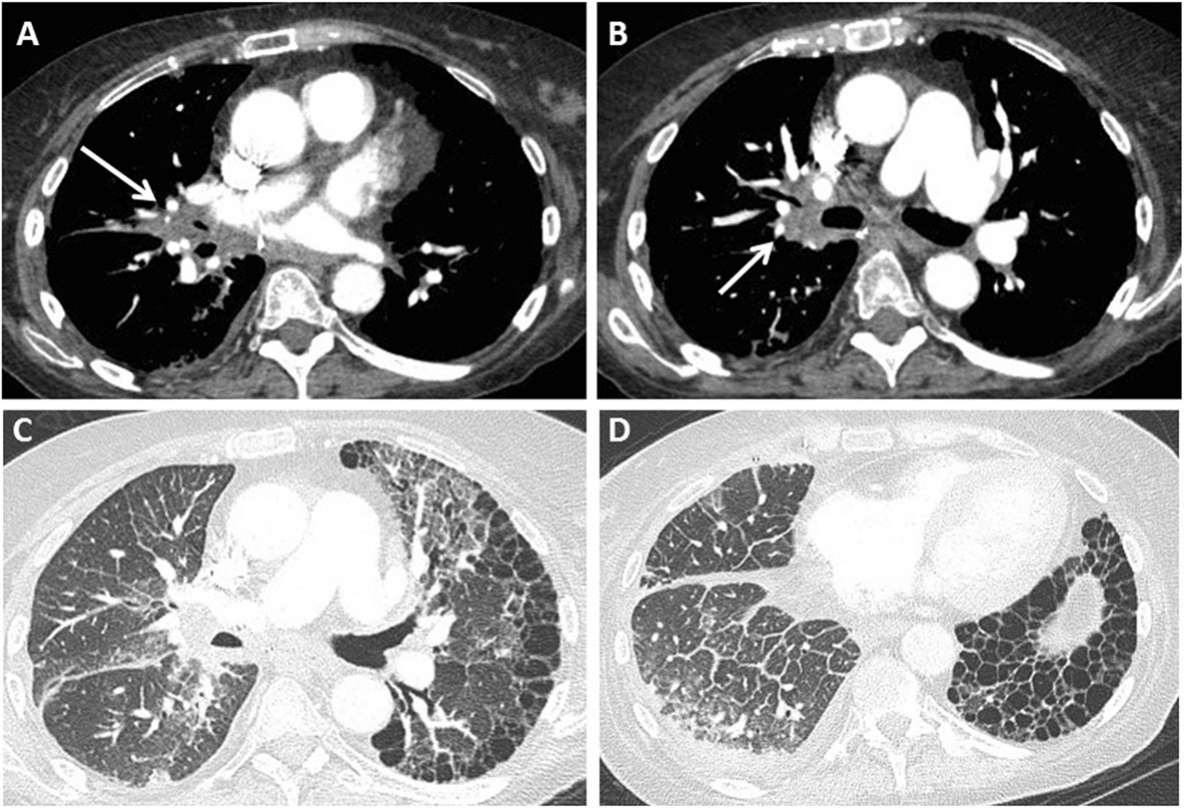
A 60-year-old male with rheumatoid arthritis–related usual interstitial pneumonia status post right lung transplant presented with shortness of breath. CT-PE protocol was performed. Axial images using soft tissue (a and b) and lung (c and d) windows show infiltrative right hilar and perihilar soft tissue density (arrows), peribronchovascular interstitial thickening, interlobular septal thickening, and ground-glass opacities within the transplanted right lung. Advanced fibrosis with exuberant honeycombing is noted in the native left lung. These findings raised concern for post-transplant lymphoproliferative disorder. Pathology and EBV PCR were negative. Flow cytometry was positive for donor specific antibody to DQ2. These findings were suggestive of antibody-mediated rejection
